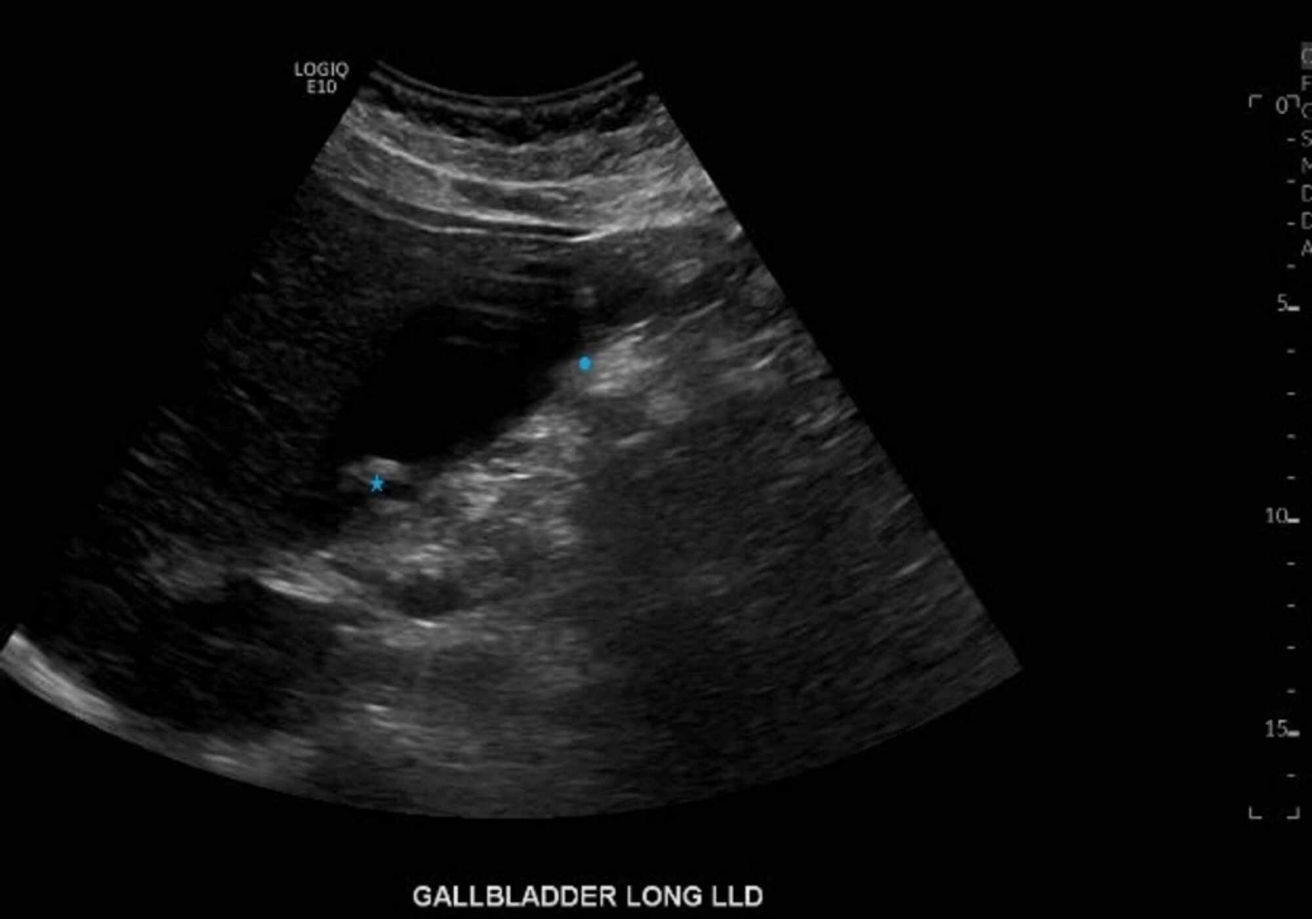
Ultrasound of the abdomen demonstrating multiple stones in the gallbladder (star) with mild prominence of the gallbladder wall (circle) concerning for acute cholecystitis
